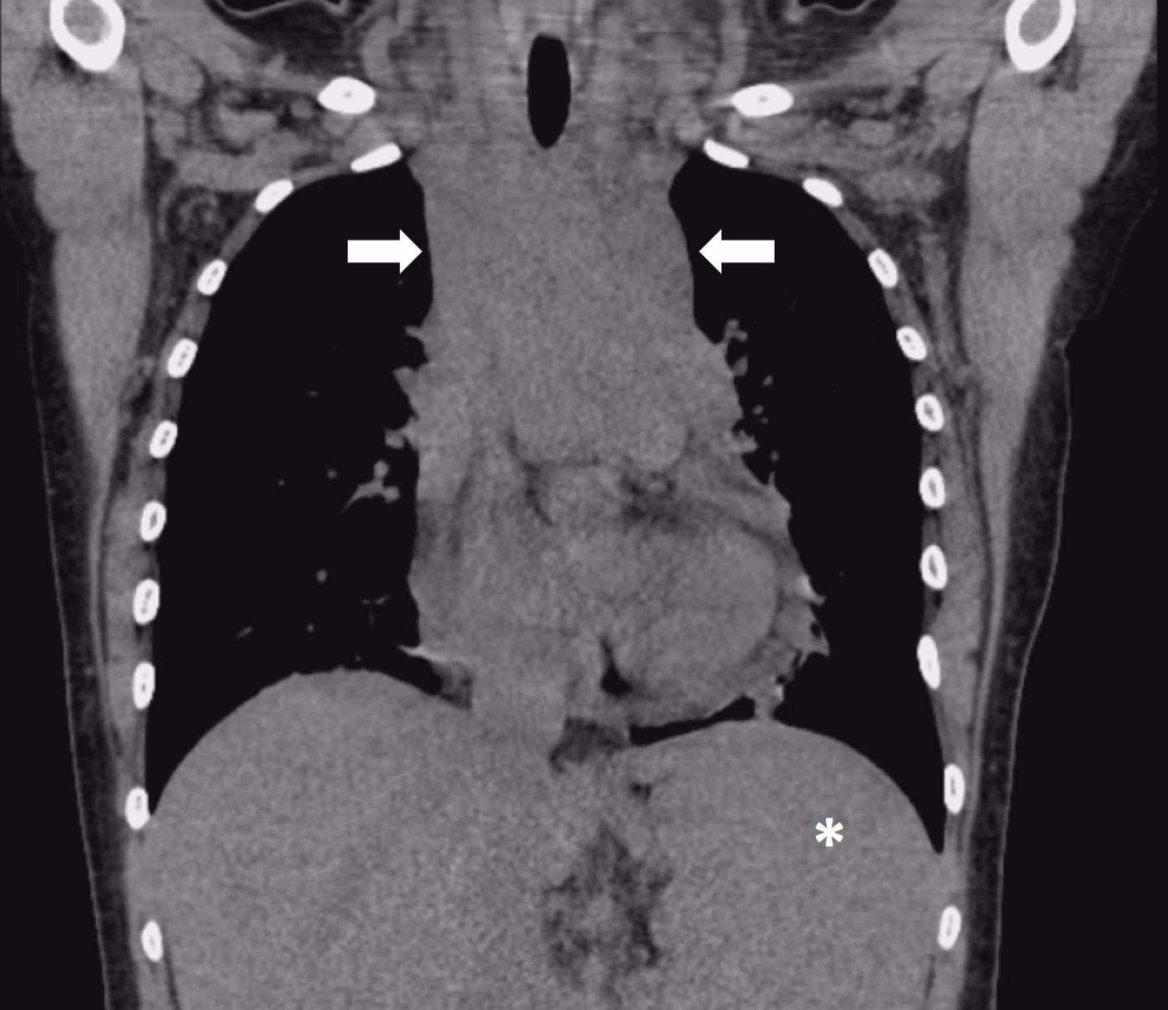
Non-contrast computed tomography with coronal view demonstrating a mass-like lobulated anterior mediastinal soft tissue density (white arrows) consistent with lymphoma and splenomegaly (asterisk).
